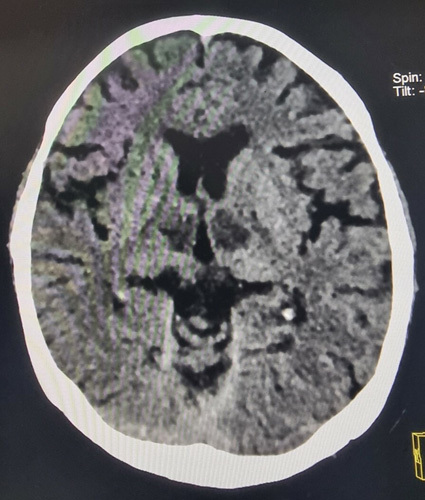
A plain computed tomographicscan of the head showing a hypodense lesion involving bilateral thalami and the rostral midbrain.
